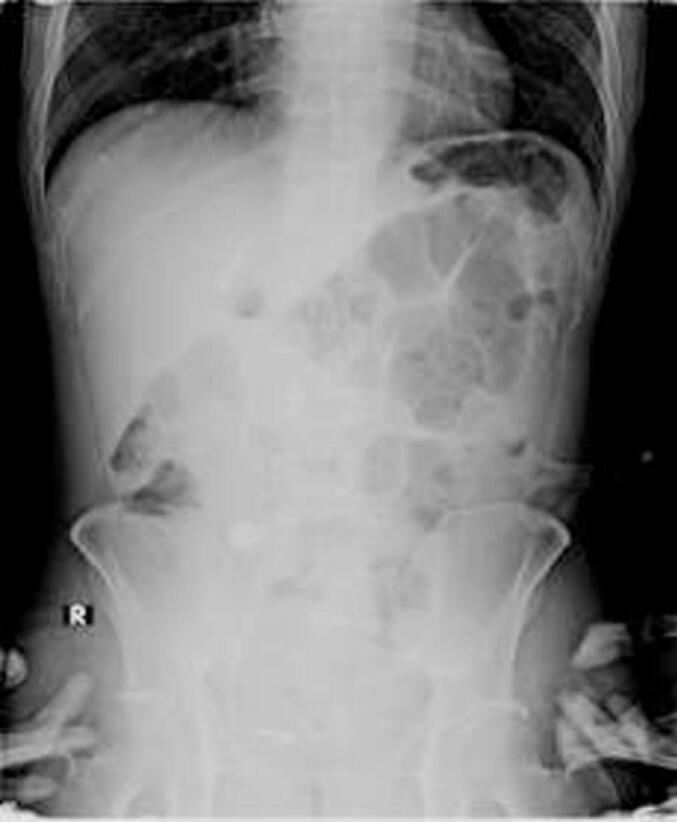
Postoperative abdominal plain radiograph: The proximal tip of the DJ stent is located near the right L1 vertebra, while the distal tip is in the pelvic cavity. A radiopaque oval-shaped shadow is observed near the right L5 vertebra, consistent with a right ureteral stone.
